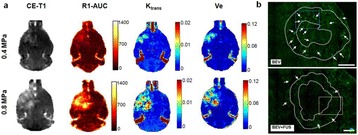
a Representative imaging indexes obtained from DCE -MRI analysis (T1-WI, R1-AUC, Ktrans, and Ve). b CD-31 IHC fluorescent microcopies. Bar = 100 μm
